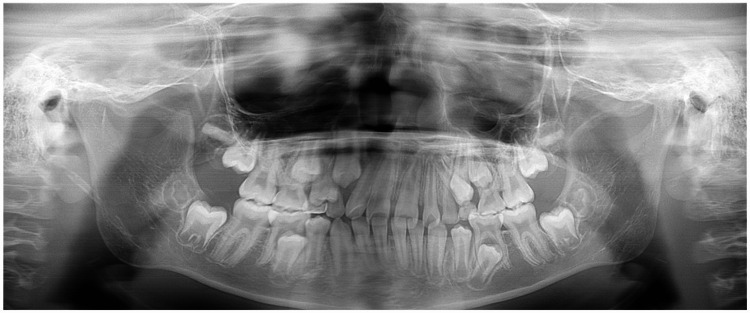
Panoramic radiograph of the younger brother with SDS The panoramic radiograph showed an ectopic position of the premolars on the left side within the alveolar bone in both the maxilla and mandible. SDS: Shwachman–Diamond Syndrome
